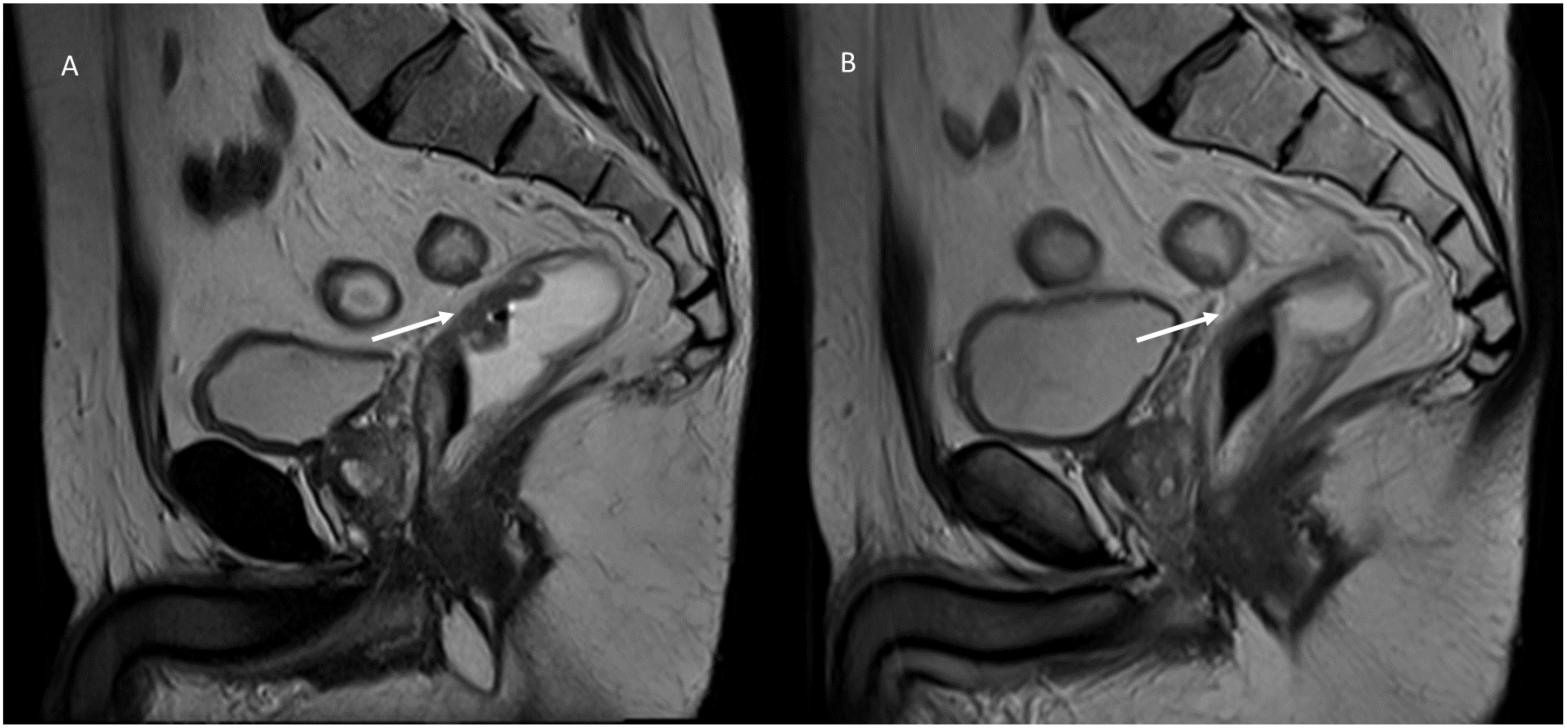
MRI assessment of post-radiotherapy fibrotic response in a patient with rectal cancer. Sagittal T2-weighted MRI images of a patient with T2-stage rectal cancer before and after short-course radiotherapy: (A) (Pre-Treatment MRI): The baseline T2-weighted sagittal image shows a well-defined, hyperintense rectal lesion (white arrow) in the mid-rectum; the lesion is infiltrating the rectal wall. (B) (Post-Treatment MRI): Follow-up MRI after short-course radiotherapy reveals a marked fibrotic response (white arrow). The lesion demonstrates a hypointense signal on T2-weighted imaging, with loss of the previously seen high signal intensity. The lesion’s borders have become less distinct, blending with the surrounding rectal wall, suggesting a treatment response with fibrosis replacing viable tumor tissue. From Vanzulli and Albano, (42).
